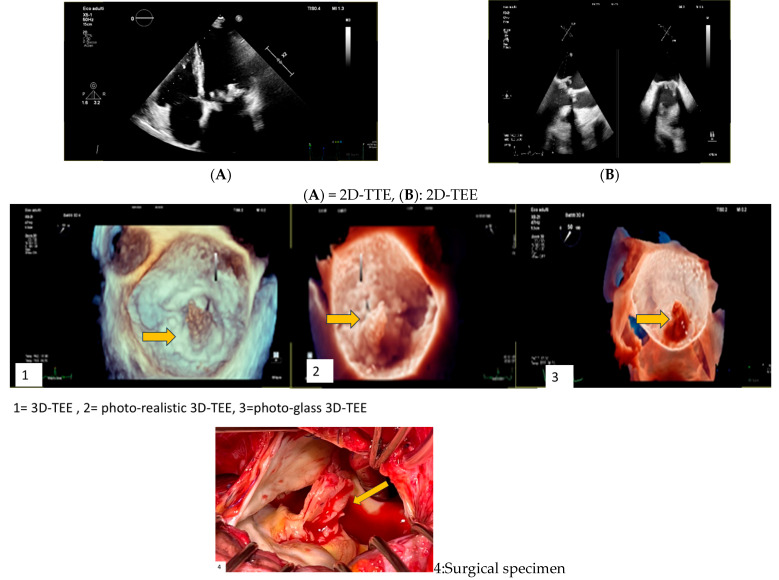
A subtle clinical phenotype of IE in elderly or frail patients deserves particular attention. Elderly patients have different risk factors, clinical presentations, and outcomes when compared with a younger population [82,83,84,85,86,87,88,89]. The classic clinical features of IE may be less prominent or even absent. The clinical picture may be characterized by nonspecific subtle symptoms, such as fatigue, confusion, or cognitive decline, making IE diagnosis challenging. Pre-existing cardiac risk conditions may be absent, and IE may overlap mild valve damage particularly caused by highly virulent bacterial healthcare-related contamination. Sometimes, frail elderly patients may show a dominant clinical feature of an explained isolated congestive heart failure before IE recognition [86]. The most common causative bacteria may include streptococcal species and healthcare-associated infections, such as *S. aureus* and enterococci. A high risk of complication may characterize the clinical presentation, with heart failure, systemic embolism, and stroke, which can open up the clinical scenario of IE. Diagnostic tests, such as blood culture, may be confounded by concurrent infection or catheter contamination and echocardiographic signs may be masked by redundant valve calcifications. The decision to operate is challenged by the presence of higher surgical risks and comorbidities. Preventive measures, such as maintaining good dental hygiene and the prompt treatment of any infections, are crucial. Multidisciplinary care is required. Early recognition, the prompt initiation of appropriate antibiotic therapy, and careful monitoring for complications are crucial in the management of IE in elderly and frail patients. Owing to these complexities, a tailored approach to a patient’s overall health status, comorbidities, and personal preferences is essential.
